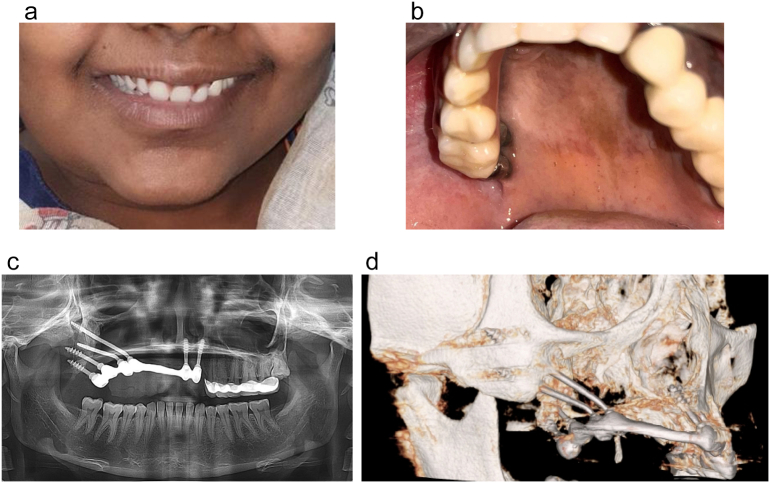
The patient's follow-up after 3 years of follow-up A. An extraoral view of the patient. B. The intraoral view of the patient depicting his clinical presentation exhibiting optimal peri-implant oral health kindly notes the smooth surface of the implant without plaque adherence. C. A panoramic radiograph after 3 years of function. D. A 3D Cone beam CT showing the prosthesis after 3 years of function.
